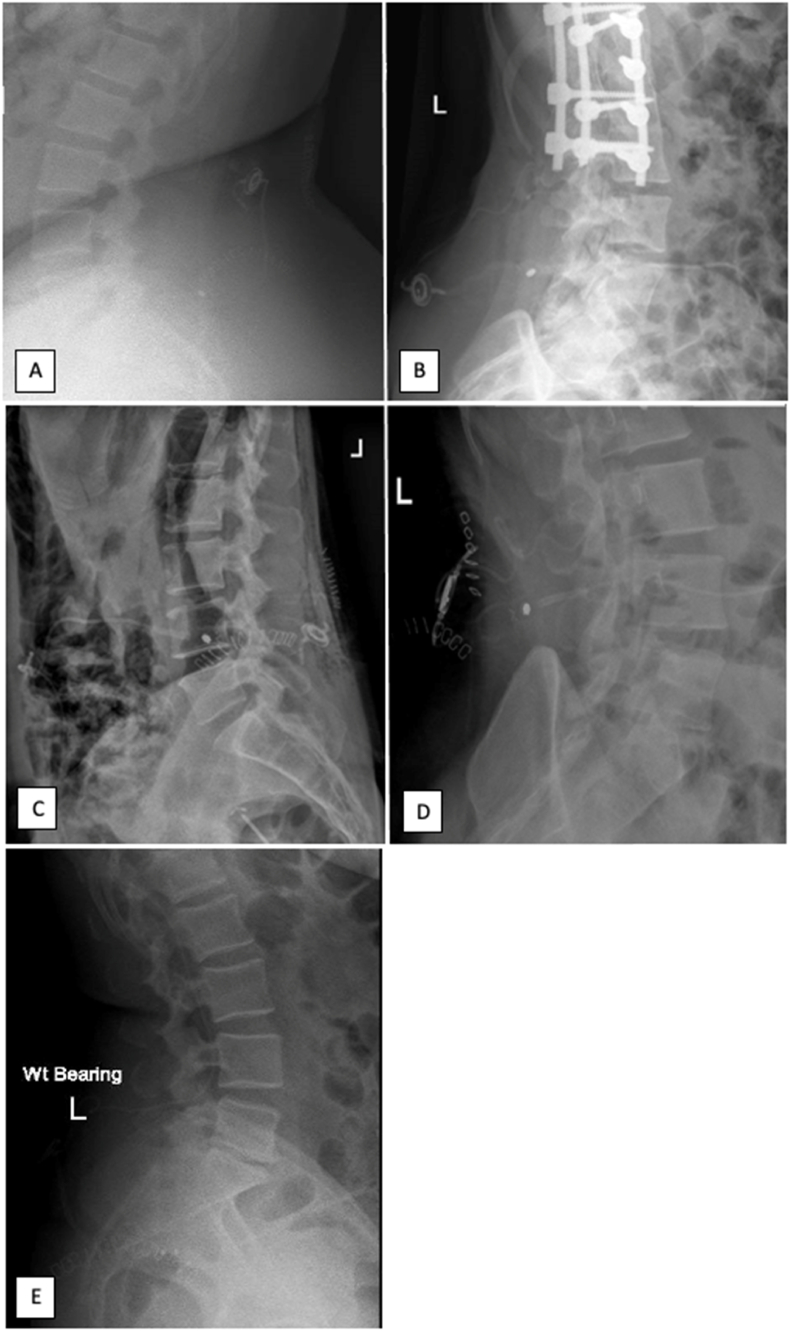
Lumbar radiographs of patients A to E, showing lumboperitoneal shunt consisting of telemetric sensor and adjustable valve.
